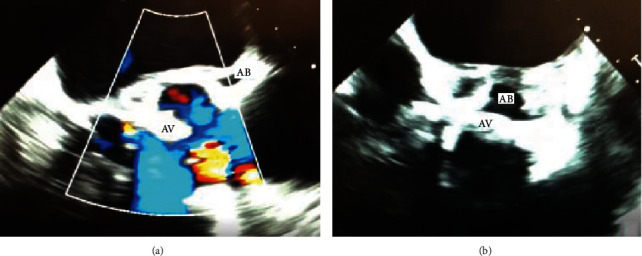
(a, b) Transesophageal echocardiogram showing aortic valve endocarditis (AV) and associated aortic root thickness and abscess. View in (a): color Doppler, apical long axis. View in (b): short-axis.
